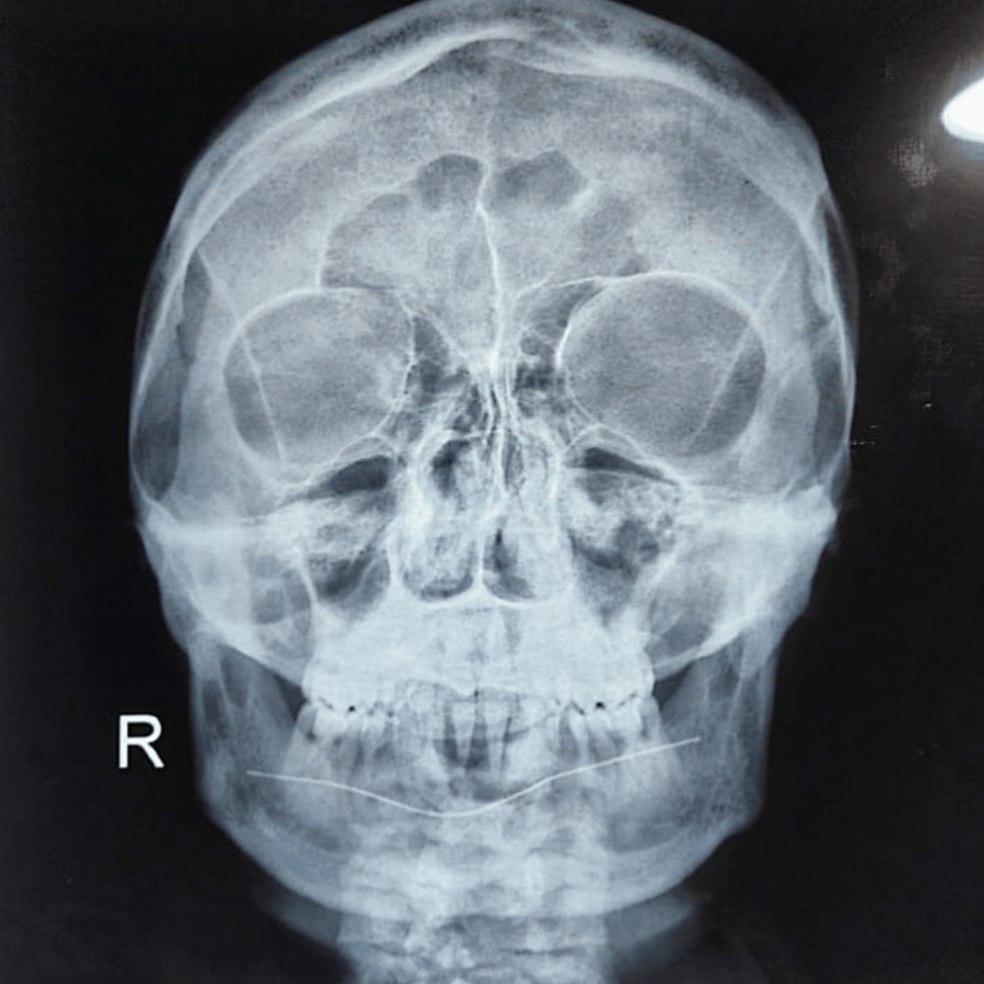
Skull X-ray showed bone expansion with widening of the calvarial diploic space and bone deformity. Alternating areas of osteolysis and osteosclerosis are suggestive of PDB.
